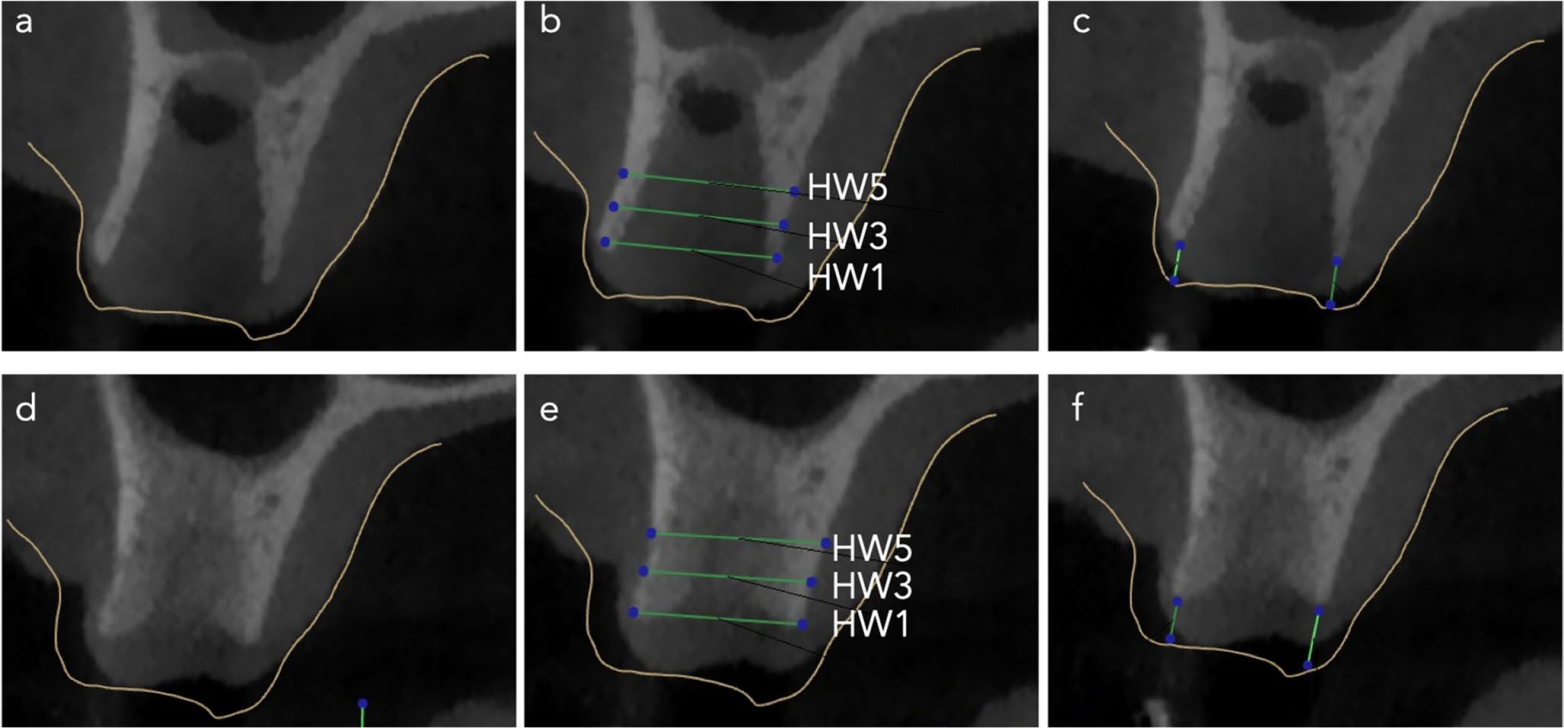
CBCT images of the extraction socket treated with PRGF for the evaluation of alveolar dimensional stability. (a) the superimposition of the STL file of the baseline cast model and the DICOM file of the baseline CBCT. (b) Baseline horizontal width measurements at 1 mm, (HW1), 3 mm (HW3) and 5 mm (HW5). (c) Baseline vertical measurement at midbuccal and mipalatal. (d) the superimposition of the STL file of the baseline cast model and the DICOM file of the 12-week CBCT. (e) 12-week horizontal width measurements at 1 mm, (HW1), 3 mm (HW3) and 5 mm (HW5). (f) 12-week vertical measurement at midbuccal and mipalatal
